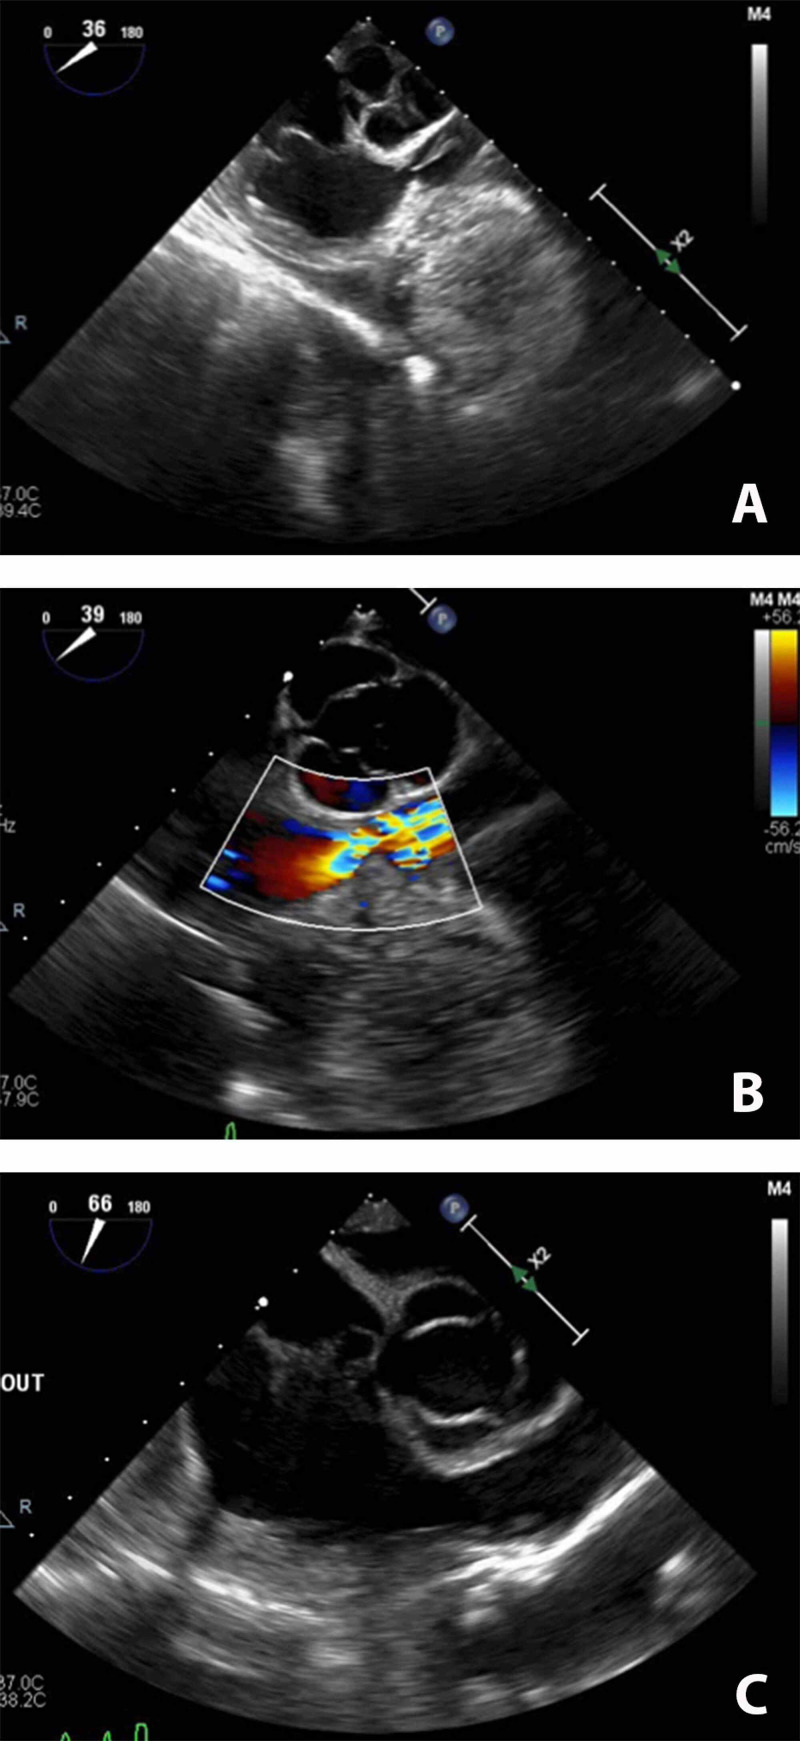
Transesophageal echocardiography imaging (A) Transesophageal echocardiography (TEE) imaging of moderate to severe right ventricular outflow tract obstruction with a pressure gradient of 55 mmHg. (B) TEE imaging of right ventricular outflow tract obstruction with color Doppler. (C) TEE imaging of the right ventricular outflow tract after resection of the mass.
